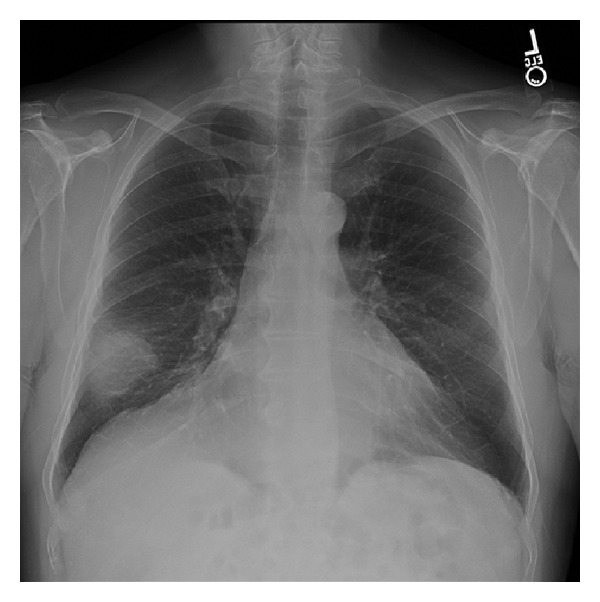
Chest radiograph demonstrating a large mass in the right middle lobe.
